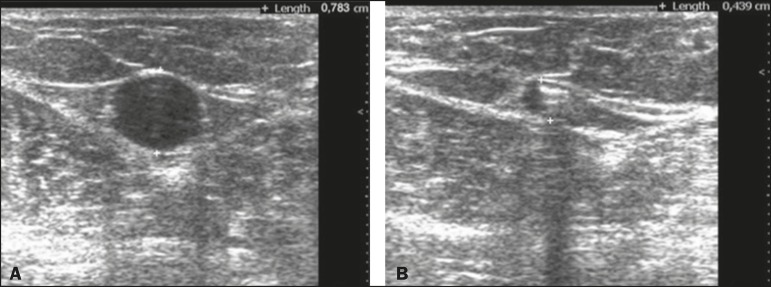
Ultrasound images of the GSV. A: Caliber of the vein before
